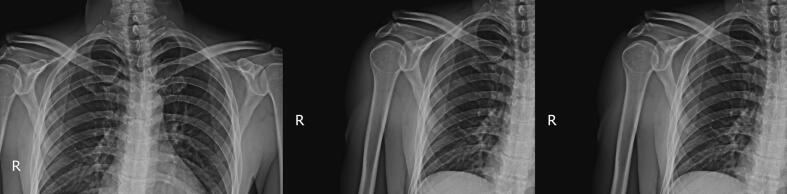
Preoperative X-ray indicating acromion fracture and AC dislocation.
